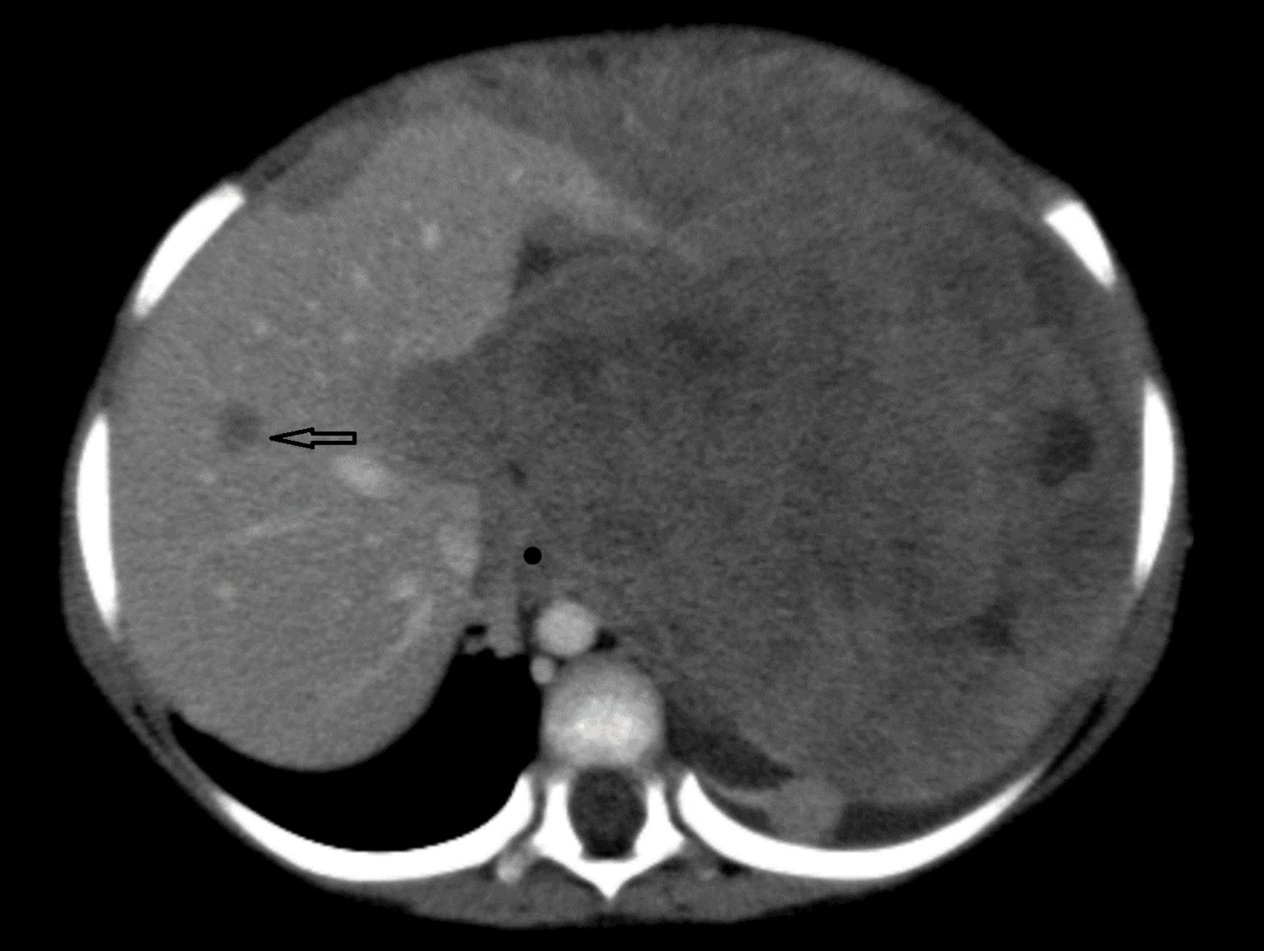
CT image (19-02-2024) showing a hepatic lesion. CT, computed tomography.
